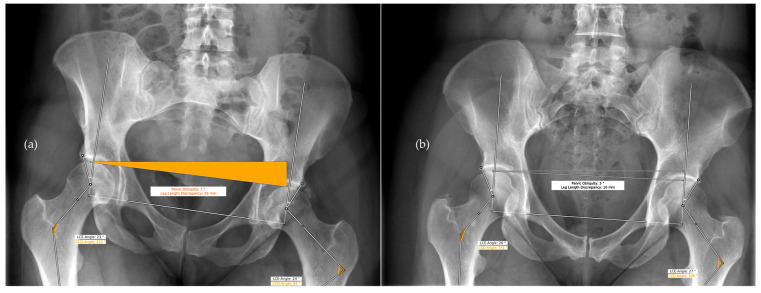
HIPPO™ erroneous reports of two AP radiographs of the pelvis that were identified as outliers. (a) Pelvic obliquity resulted in a cropped proximal femur and the anatomical femur axis could not be identified correctly. (b) The proximal femur is barely visible and the anatomical femur axis could not be identified correctly.
